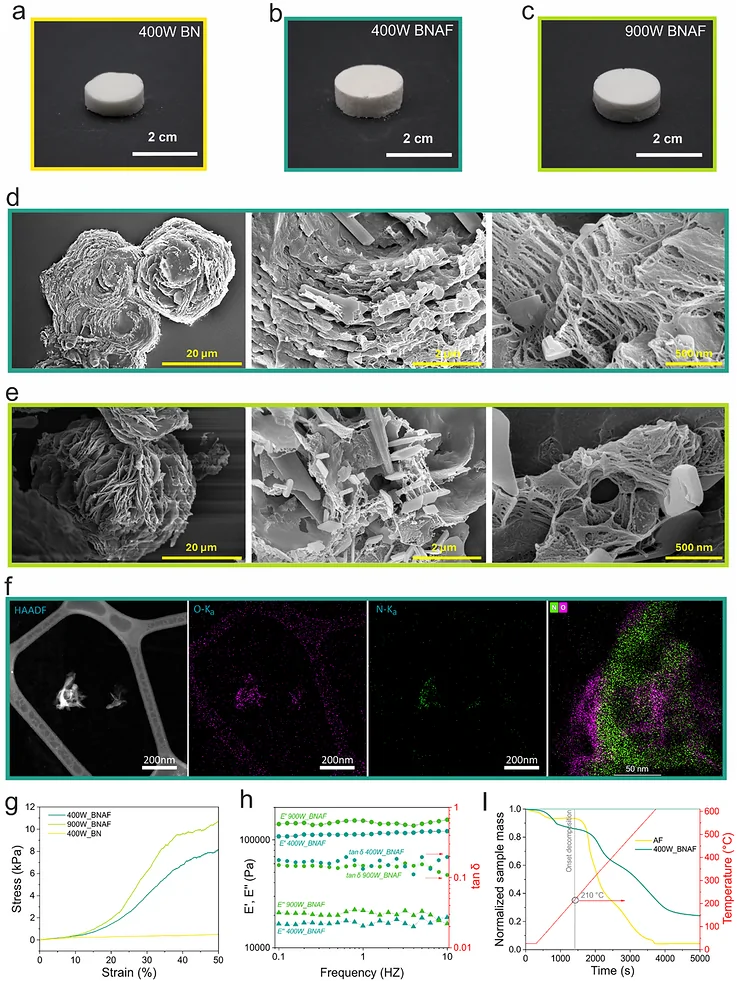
Characterization of BN and WPI amyloid fibril (BNAF) aerogels. a) Aerogel produced at 400 W ultrasonication without amyloid fibrils. b) 400 W hybrid BN–amyloid fibril aerogel. c) 900 W hybrid BN–amyloid fibril aerogel. d) Multi‐magnification SEM images of the 400 W BNAF aerogel fracture surface (scale bars: 20, 2, and 500 nm, from left to right). e) Multi‐magnification SEM images of the 900 W BNAF aerogel fracture surface (scale bars: 20, 2, and 500 nm, from left to right). f) STEM–EDS characterization of the 400 W BN–amyloid hybrid, providing local compositional support for nitrogen‐containing inorganic domains. HAADF image, O‐K and N‐K elemental maps, and drift‐corrected overlay map. g) Compression tests at 50% strain comparing the three aerogels. h) DMA showing E′, E″, and tan δ of aerogels across the frequency range sampled. i) TGA of pure amyloid fibrils and the 400 W hybrid BN–amyloid fibril aerogels.
